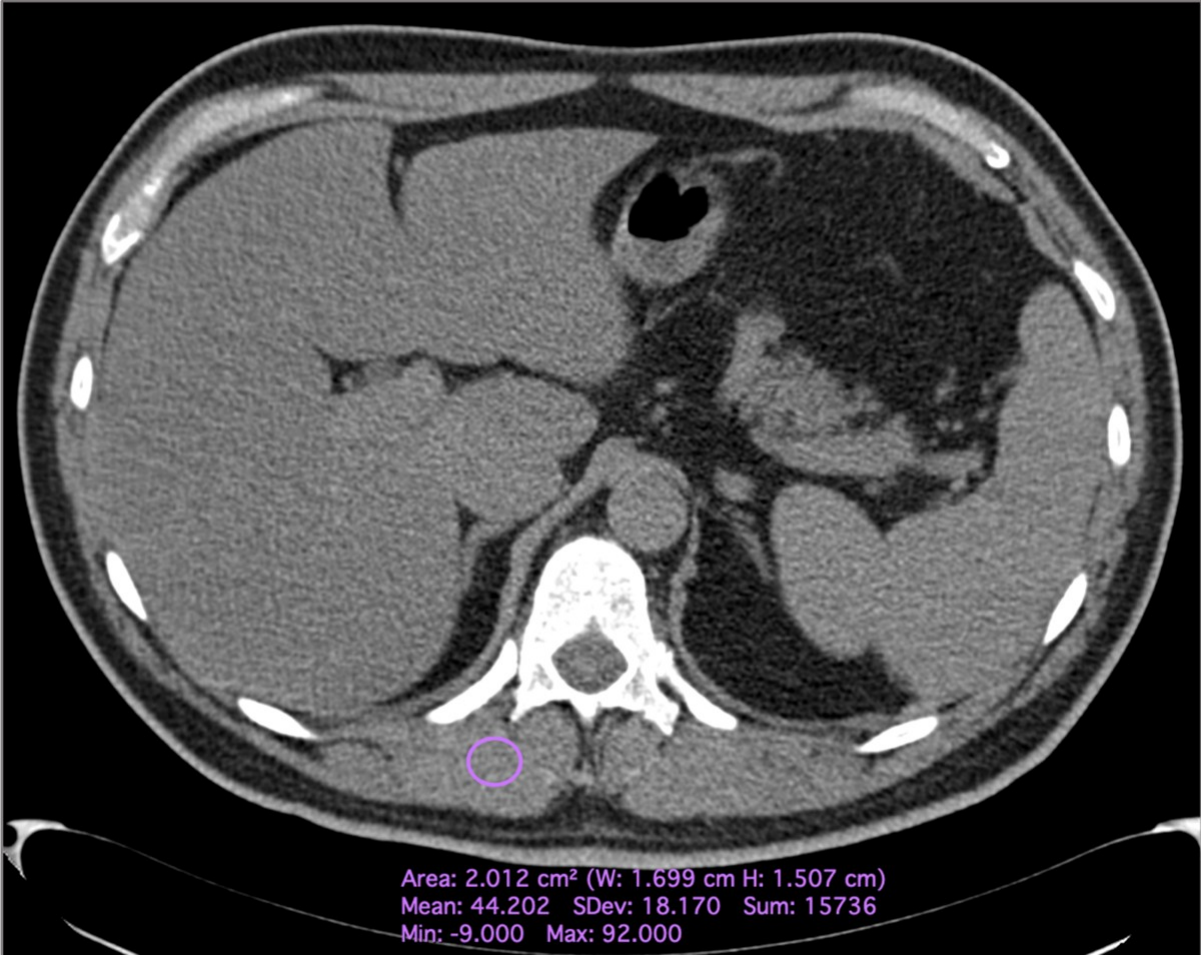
Axial Computed Tomography images of a 59 years-old male affected by COVID-19, hospitalized in a COVID-19 ward, demonstrating the applied method of measurements of muscle densitometry. A circular 2 cm size region of interest was placed on the right paravertebral muscle at the level of the 12^th^ dorsal vertebra and the mean Hounsfield unit value collected.
